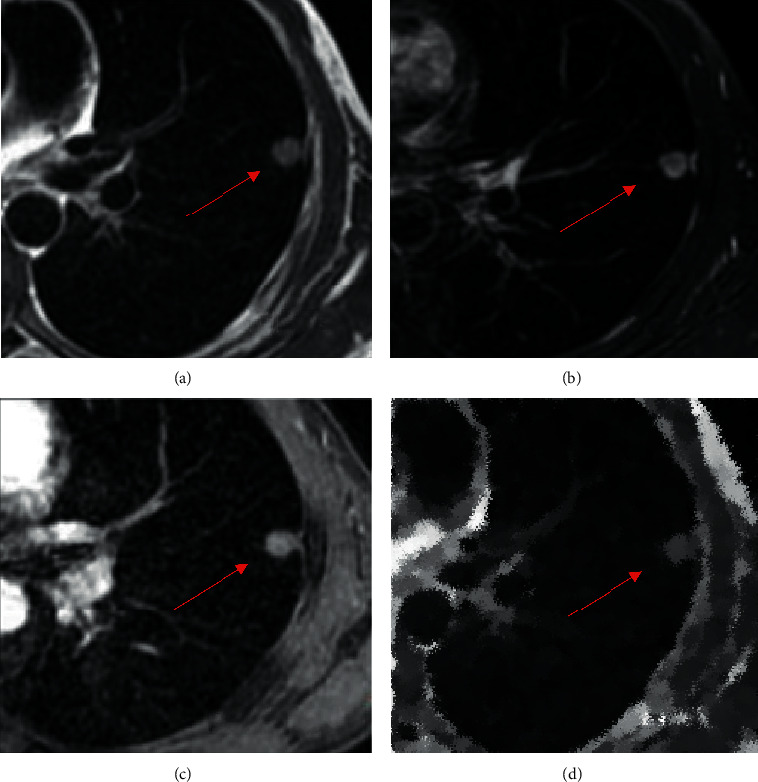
MRI features of SPN patients. (a) T1W1 image; (b) T2W1 image; (c) DW1 image; and (d) ADC image.
